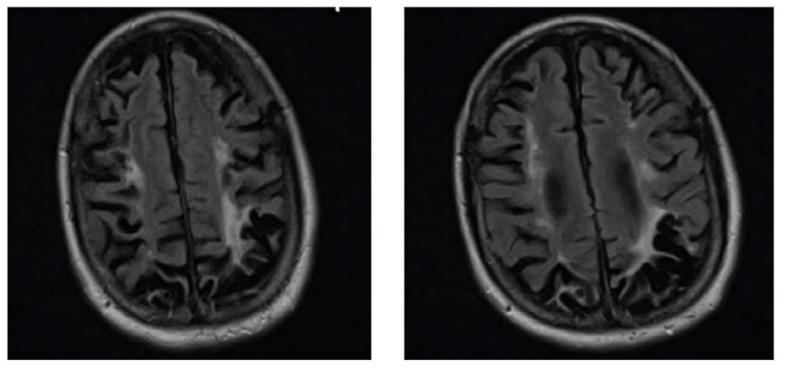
An MRI of the brain showed an increased white matter signal in the centrum semiovale representing encephalomalacia. There was generalized prominence of the cortical sulci representing diffuse cerebral volume loss.
